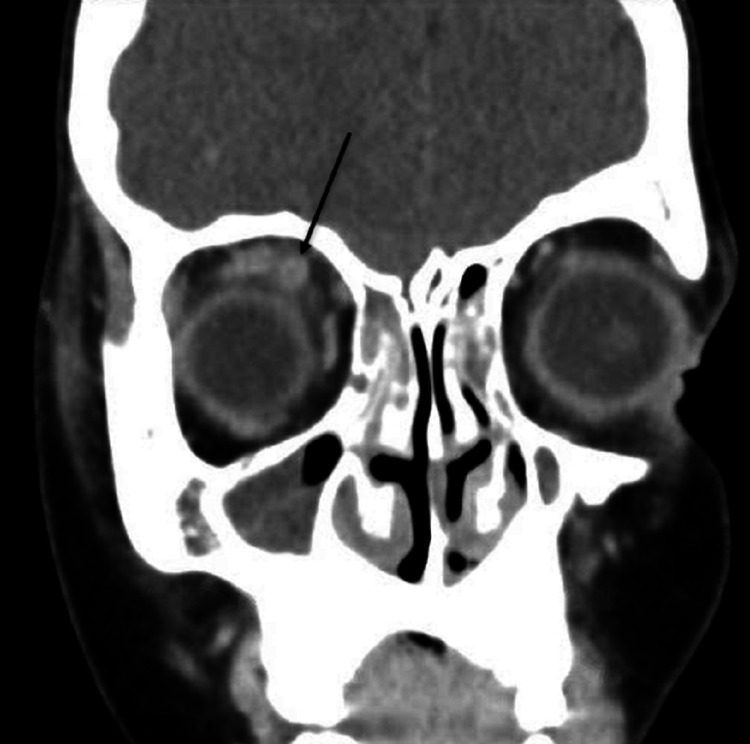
Post-contrast CT (coronal view) showing right superior ophthalmic vein thrombosis with vein enlargement in comparison to the left side with sinusitis.
